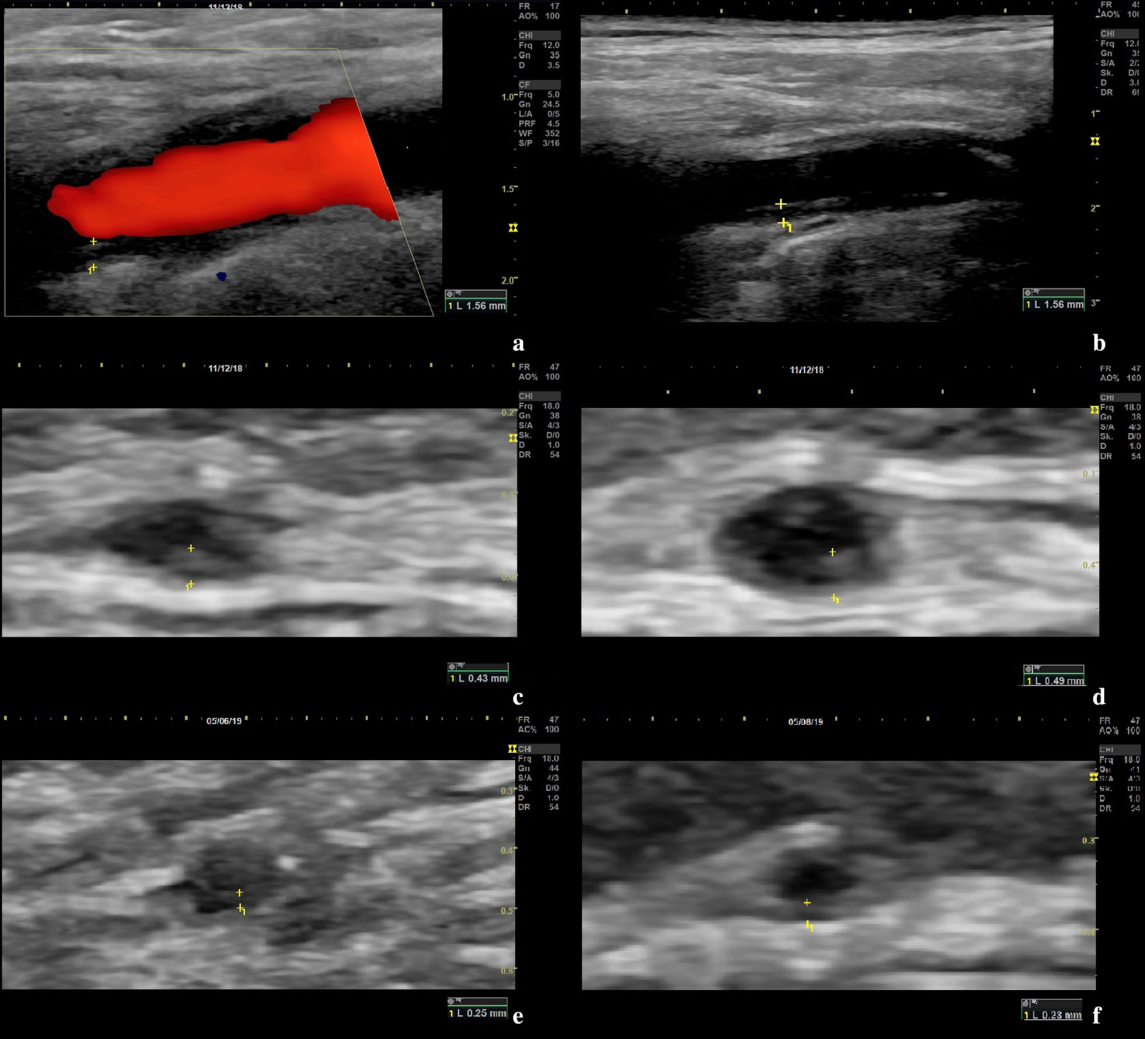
Ultrasound images displaying vasculitic changes of the intima-media complex of the respective arteries. a, b Left vertebral artery in Doppler and B-mode ultrasound with pathological vessel wall and increased intima-media thickness (IMT) due to inflammation. c Right common superficial temporal artery with pathological IMT of 0.43 mm. d Left common superficial temporal artery with pathological IMT of 0.49 mm. e Right common superficial temporal artery in 6-month follow-up with IMT of 0.25 mm. f Left common superficial temporal artery in 6-month follow-up with IMT of 0.23 mm
